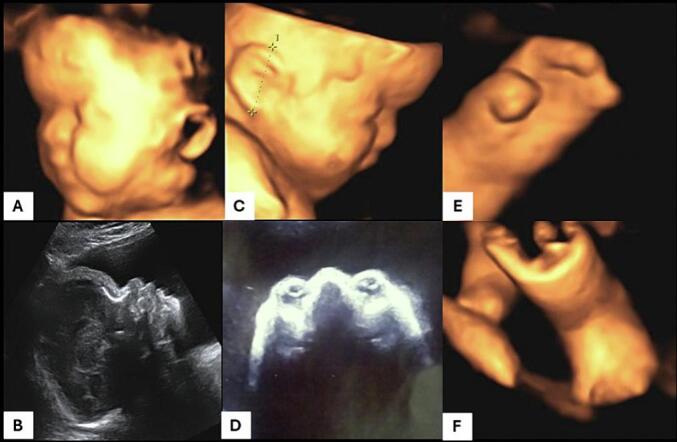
Third-trimester ultrasound findings showing midface hypoplasia with a prominent forehead in 3D (A) and 2D (B), low-set ears (C), hypertelorism, (D) bilateral syndactyly of both hands (mitten hands) appearance) (E), and deformed feet (F).
